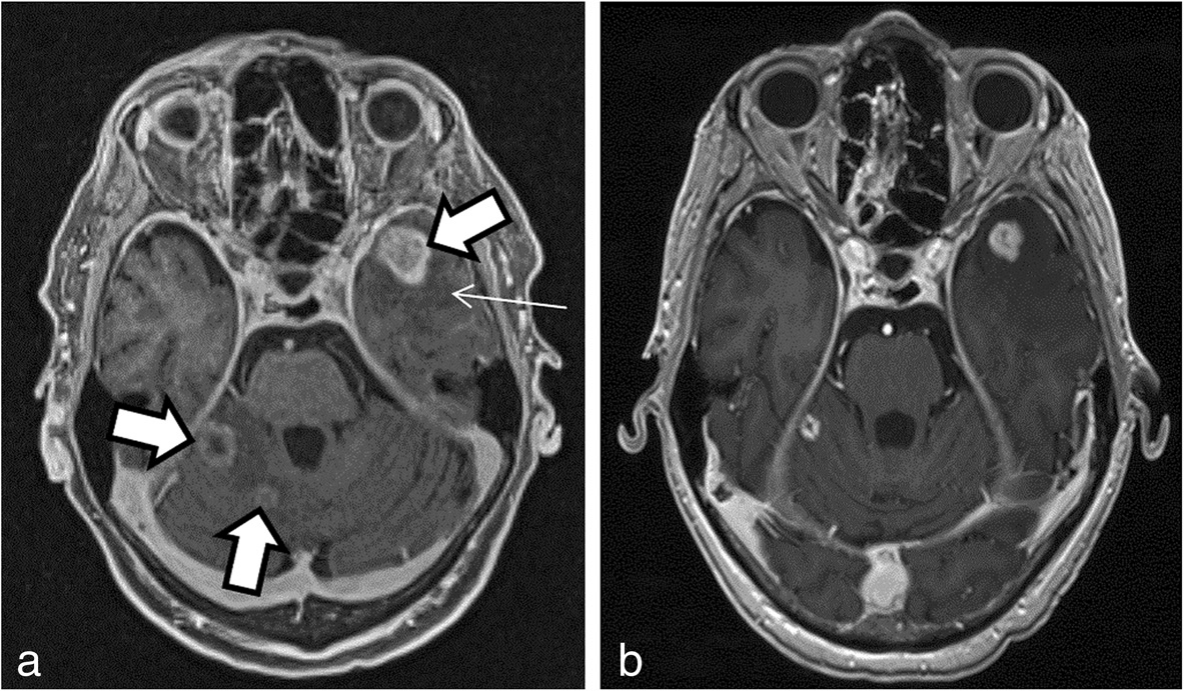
Magnetic resonance imaging of brain metastases of cholangiocarcinoma. (a) Multiple metastases of the cholangiocarcinoma were observed before radiotherapy in a T1 multiplanar reconstruction of magnetic resonance imaging sequences after intravenous injection of a gadolinium chelated contrast agent. Rounded lesions are present in the left frontal lobe and right cerebellum. The lesions show peripheral enhancement and are moderately thick and irregular. The lesion center is hypointense or nonenhancing (thick arrows). A perilesional hyposignal corresponding to perilesional edema (thin arrow) is also present. (b) Evolution of brain metastases 2 months after the end of radiotherapy, followed by chemotherapy. The lesion volume is reduced, but no changes are apparent in the enhancement features or in the perilesional edema.
